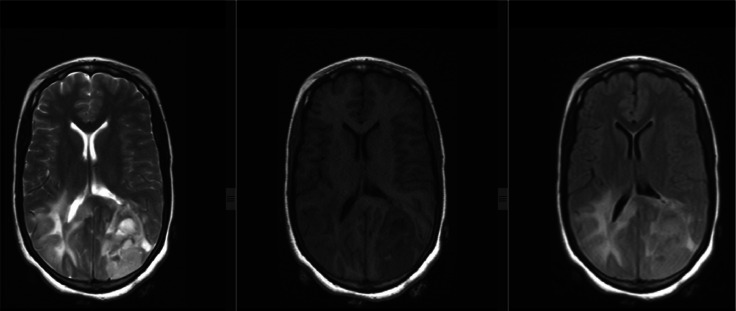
MRI images of PCNSL. From left to right, T2 hyperintense, T1 hypointense, and FLAIR hyperintense subcortical and cortical lesions involving the bilateral occipito-parietal lobe, temporal lobe, and parietal lobes.
